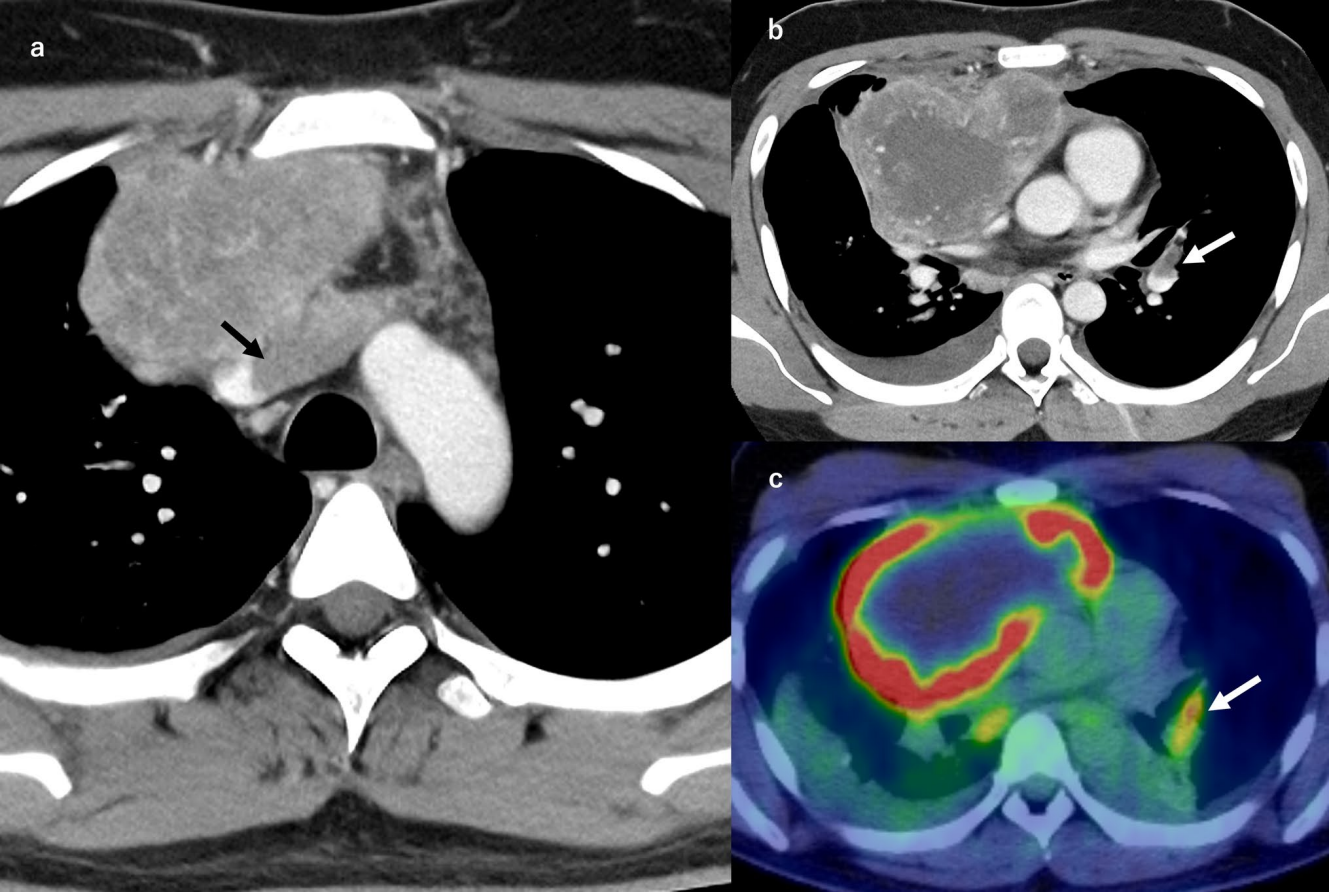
Macroscopic pulmonary tumor embolism (PTE). A man in his 30 s was diagnosed with a macroscopic PTE originating from an anterior mediastinal germ cell tumor. Contrast-enhanced CT revealed a large anterior mediastinal mass with heterogeneous internal enhancement (a, b) and significant FDG uptake (SUVmax 19.72) (c). The mass was seen infiltrating the left brachiocephalic vein (a). A tumor embolus with faint contrast enhancement was also noted in the left pulmonary artery (b). This intravascular lesion also demonstrated FDG accumulation (SUVmax 5.66), consistent with a tumor embolism (c)
